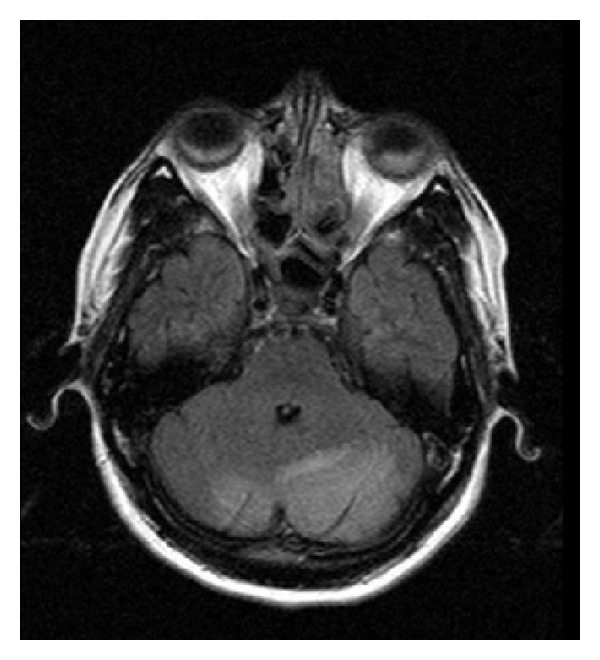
MRI: hyperintense signal involving the white matter typically in occipital regions.
